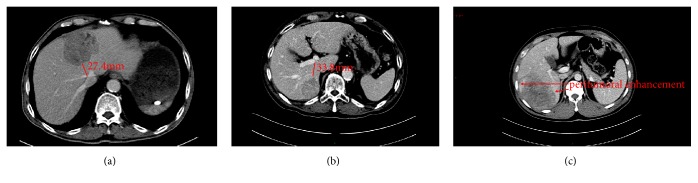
Typical CT images of patients with hepatocellular carcinoma.  Figure 1(a) shows the distance from the tumor to the IVC. The closest distance from the tumor to the inferior vena cava on the cross section of the venous-phase image was selected.  Figure 1(b) shows the distance from the tumor to the portal vein branches. When measuring the distance from the tumor to the portal vein branches, the portal vein branches were first positioned on the transverse section. Then, the shortest line from the portal vein branches to the tumor was drawn. As shown in Figure 1(c), we detected arterial-enhanced portions adjacent to the tumor border on arterial-phase images that became isodense with background liver parenchyma on delayed-phase images.
